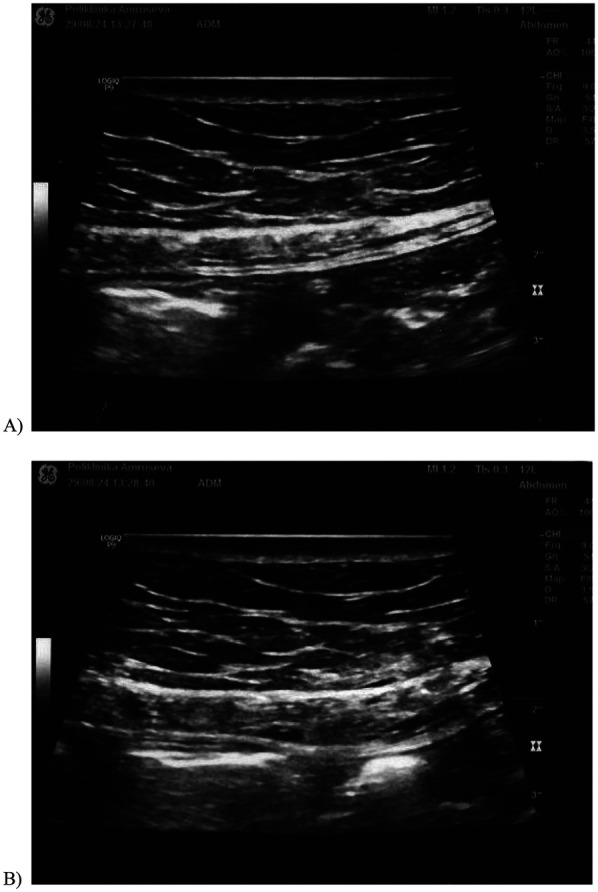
The ultrasound image of the abdominal wall: (A) before the block of the anterior cutaneous nerve and (B) after the block of the anterior cutaneous nerve.
